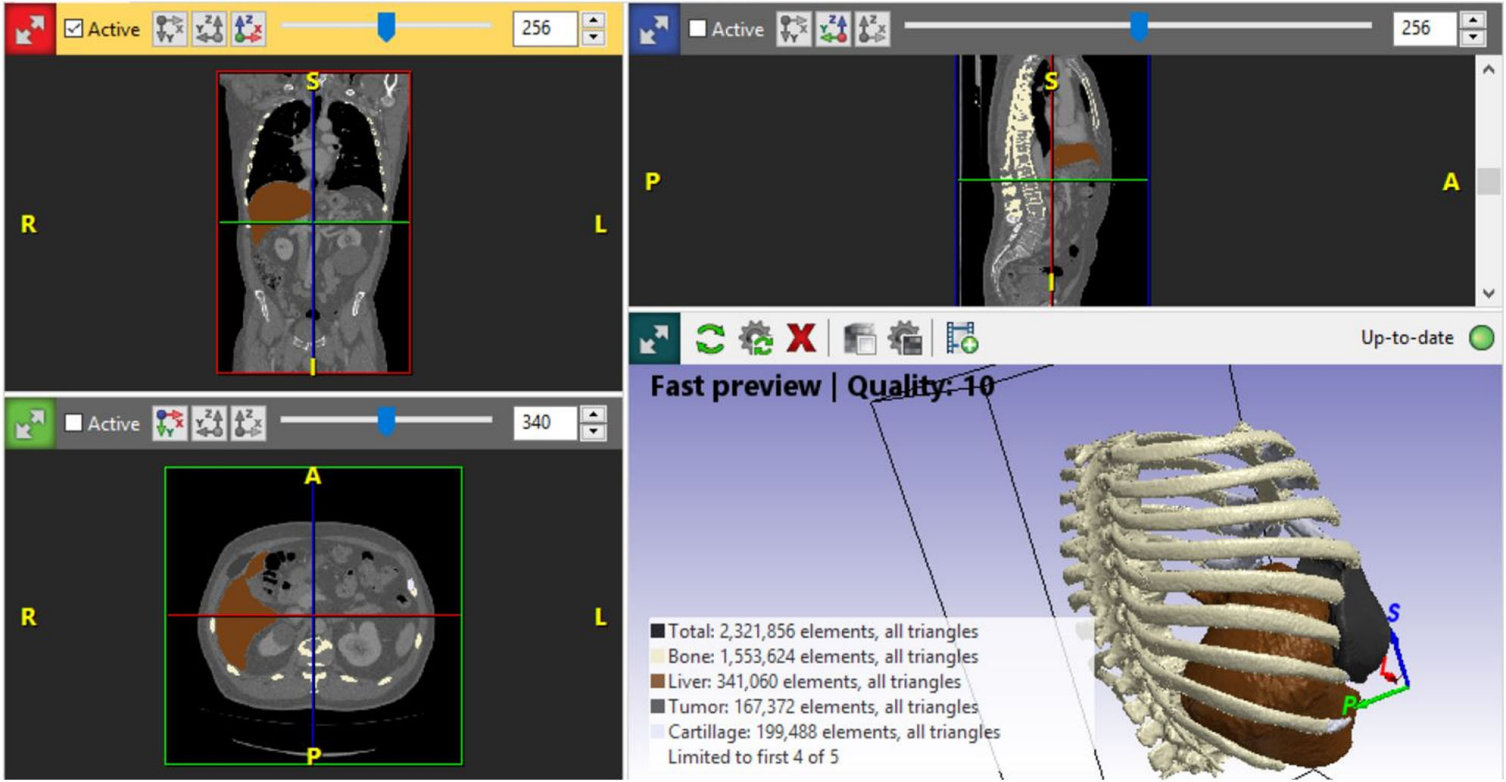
Simpleware ScanIP software snapshot applied for segmentation of the organ, ribs, cartilages, and tumor. ScanIP is the software used for comprehensively processing three-dimensional image data from a computed tomography scan. The software provides powerful image visualization, analysis, segmentation, and quantifications tools.
